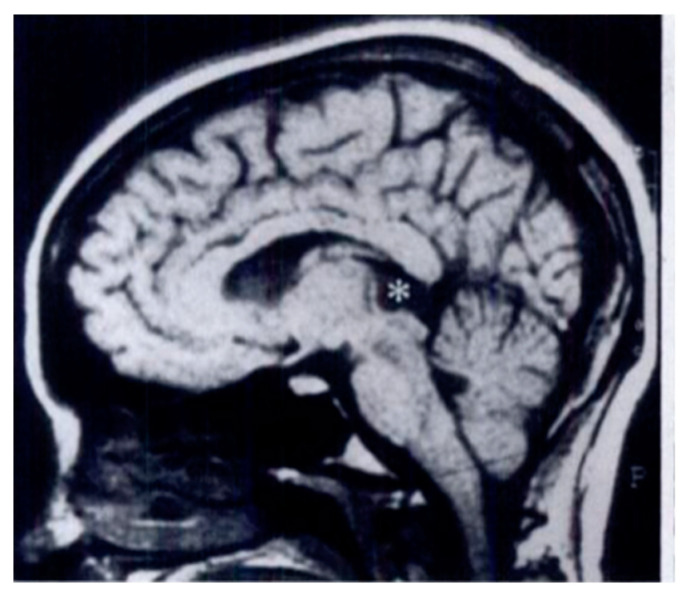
Pineocytoma MRI. The asterisk indicates the homogeneous mass in the pineal gland. Reprinted with permission from Smirniotopoulos et al. (1992) [13]. Radiological Society North America–2021. Small pineocytomas often do not induce symptoms, but if they are of large dimensions, they may induce obstructive hydrocephalus and Parinaud syndrome, defined as upward gaze palsy, pupillary light-near dissociation, and convergence retraction nystagmus [15]. The five- and twenty-year survival rates are, respectively, 100% and 76% [21,26]. For pineocytomas, stereotactic radiosurgery seems to be highly effective as a primary treatment, so stereotactic radiosurgery alone may be considered appropriate clinical management for pineocytomas [21,28,71,72].
